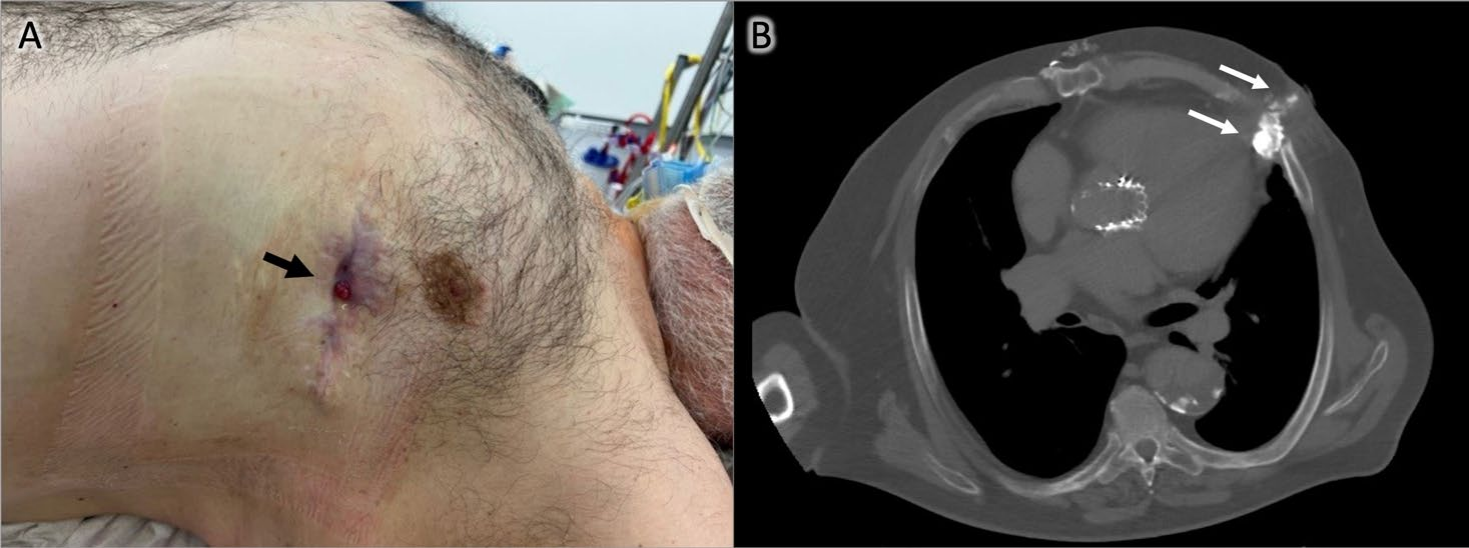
(A) Chronic fistula of the left lateral thorax; (B) CT scan after contrast injection through the chronic wound, showing a fistula reaching the cardiac apex (white arrows).
